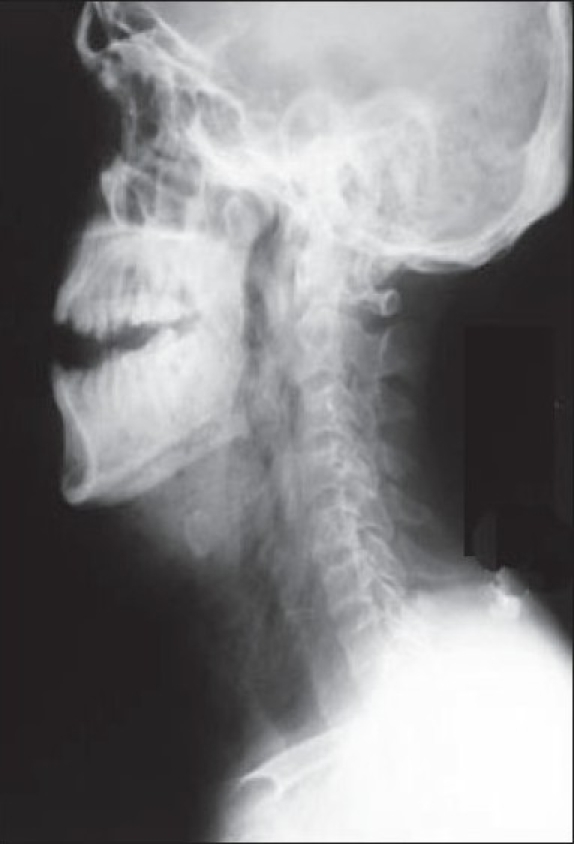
X-ray showing presence of subcutaneous gas formation
